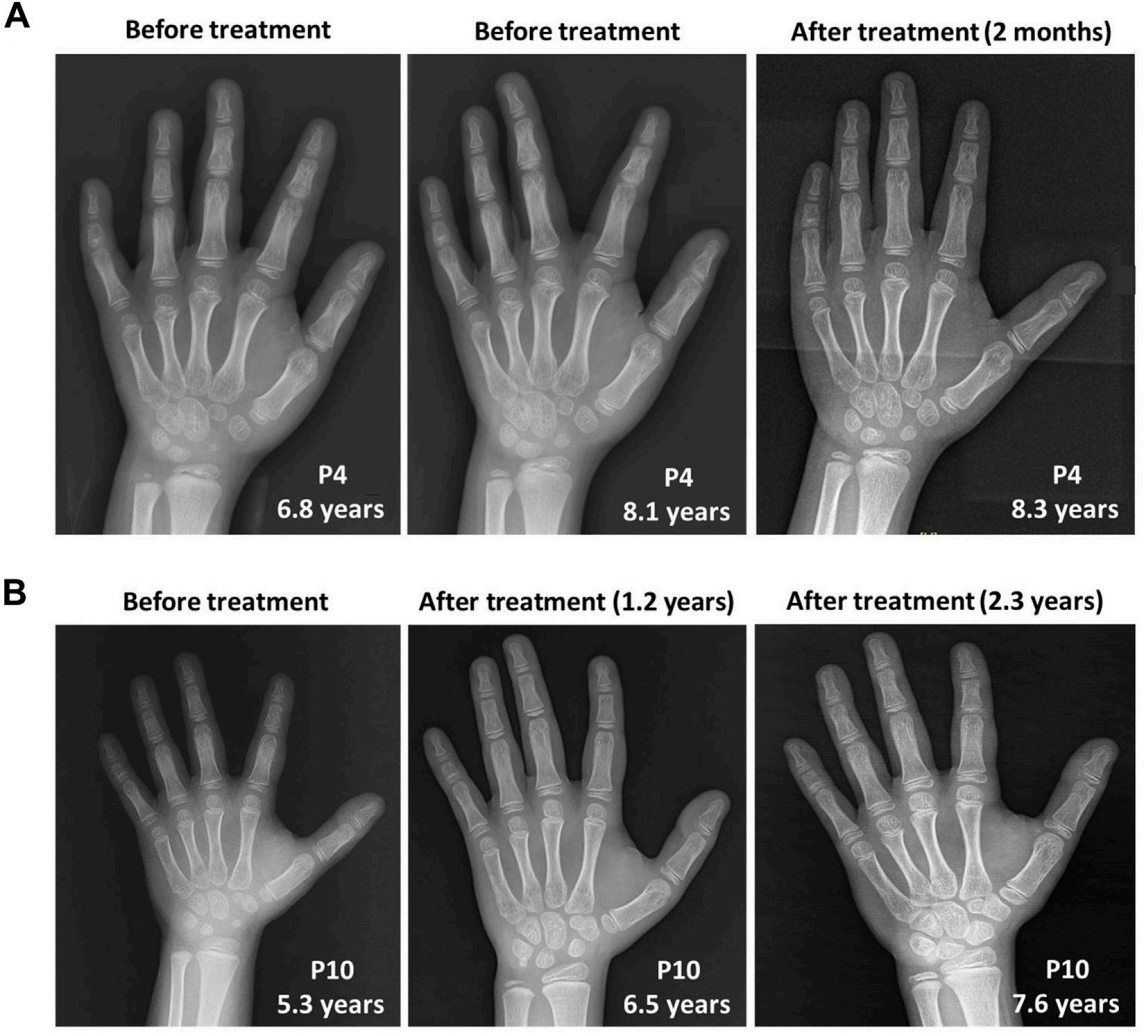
The skeletal X-ray images of two WSS patients before and after rhGH treatment. (A) P4. (B) P10.
